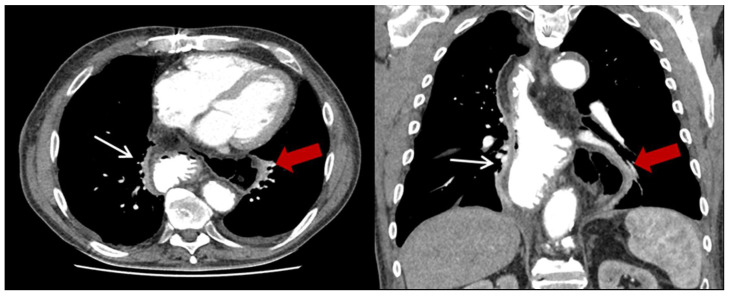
Exemplary CT-scan of a hiatal hernia on the left thoracic side after HMIE. Gastric conduit marked with thin white arrow; herniated colon marked with thick red arrow.
